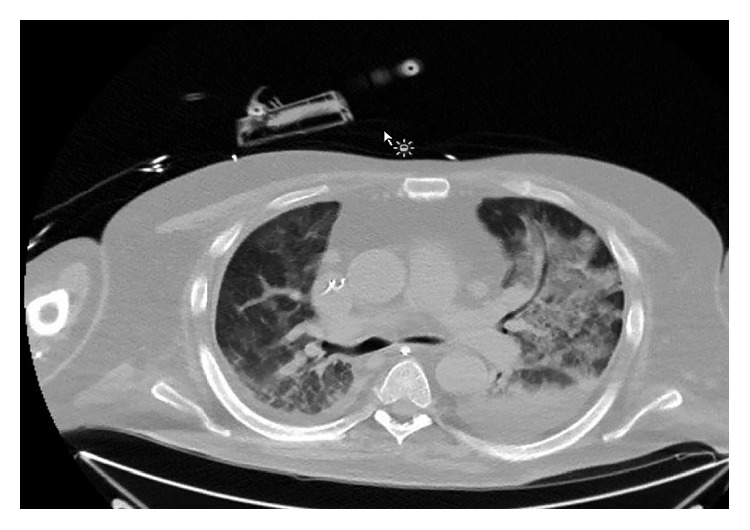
CT scan of the chest showing multifocal airspace disease concerning pneumonia or oedema.
